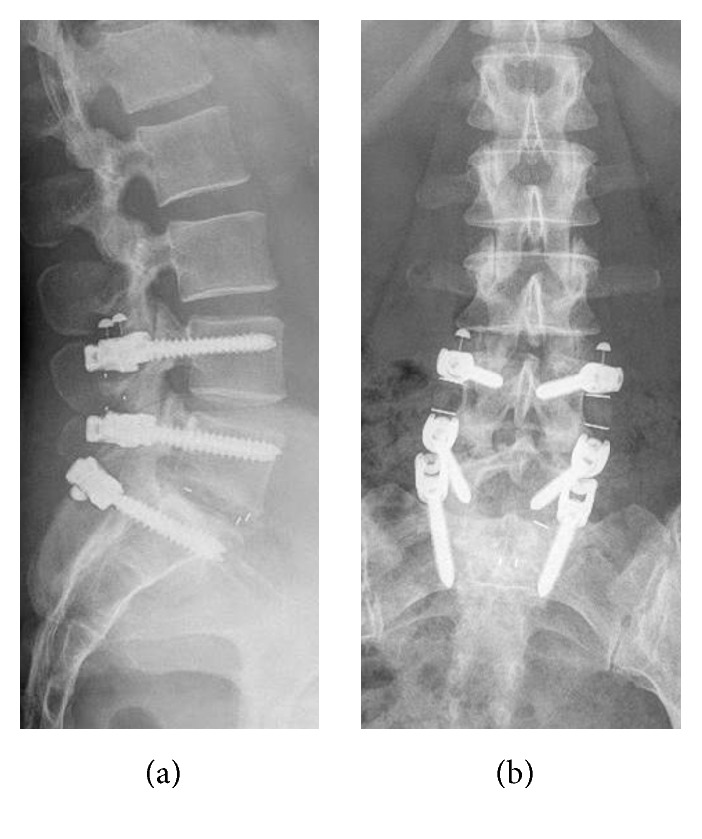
Sagittal (a) and AP (b) radiograph of the lumbar spine in a standing position after hybrid instrumentation with BalanC.
